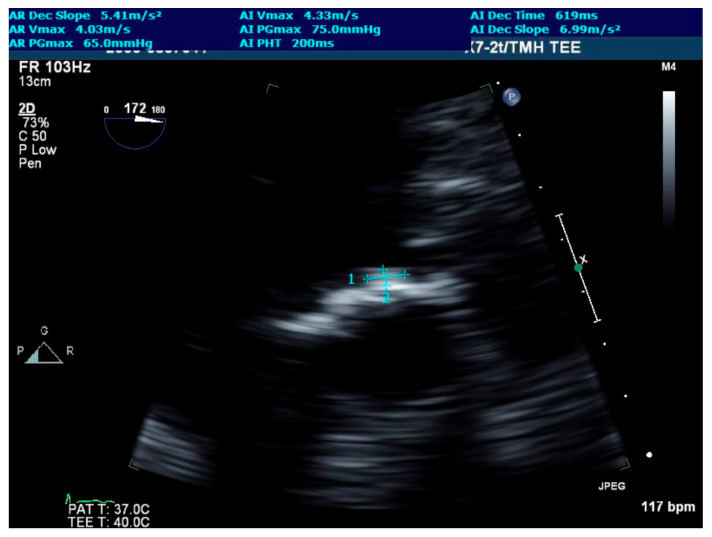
Zoomed-in transesophageal echocardiogram deep transgastric view of the aortic valve from the left ventricular outflow tract. Cross-hairs depict the mass on the aortic valve.
